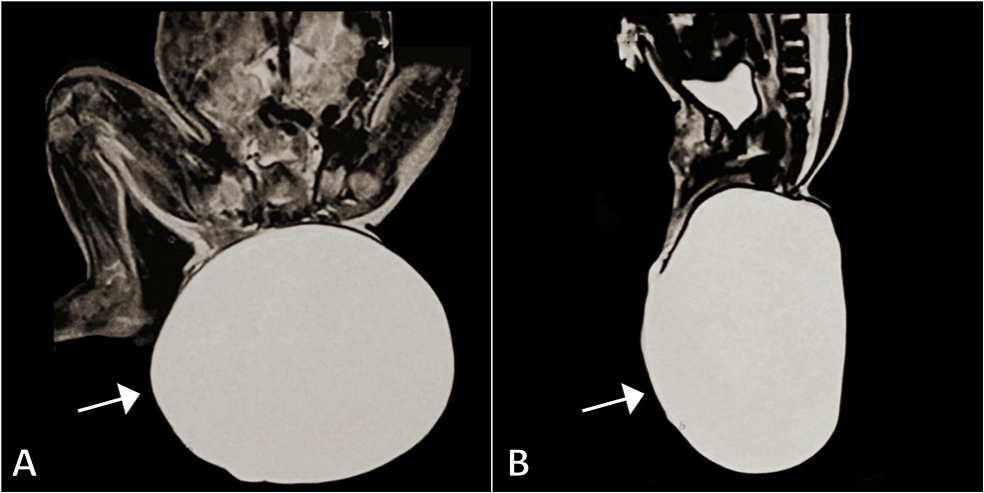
Coronal (A) and sagittal (B) MR images showing a large cystic sacrococcygeal lesion (arrows). MR: Magnetic resonance
